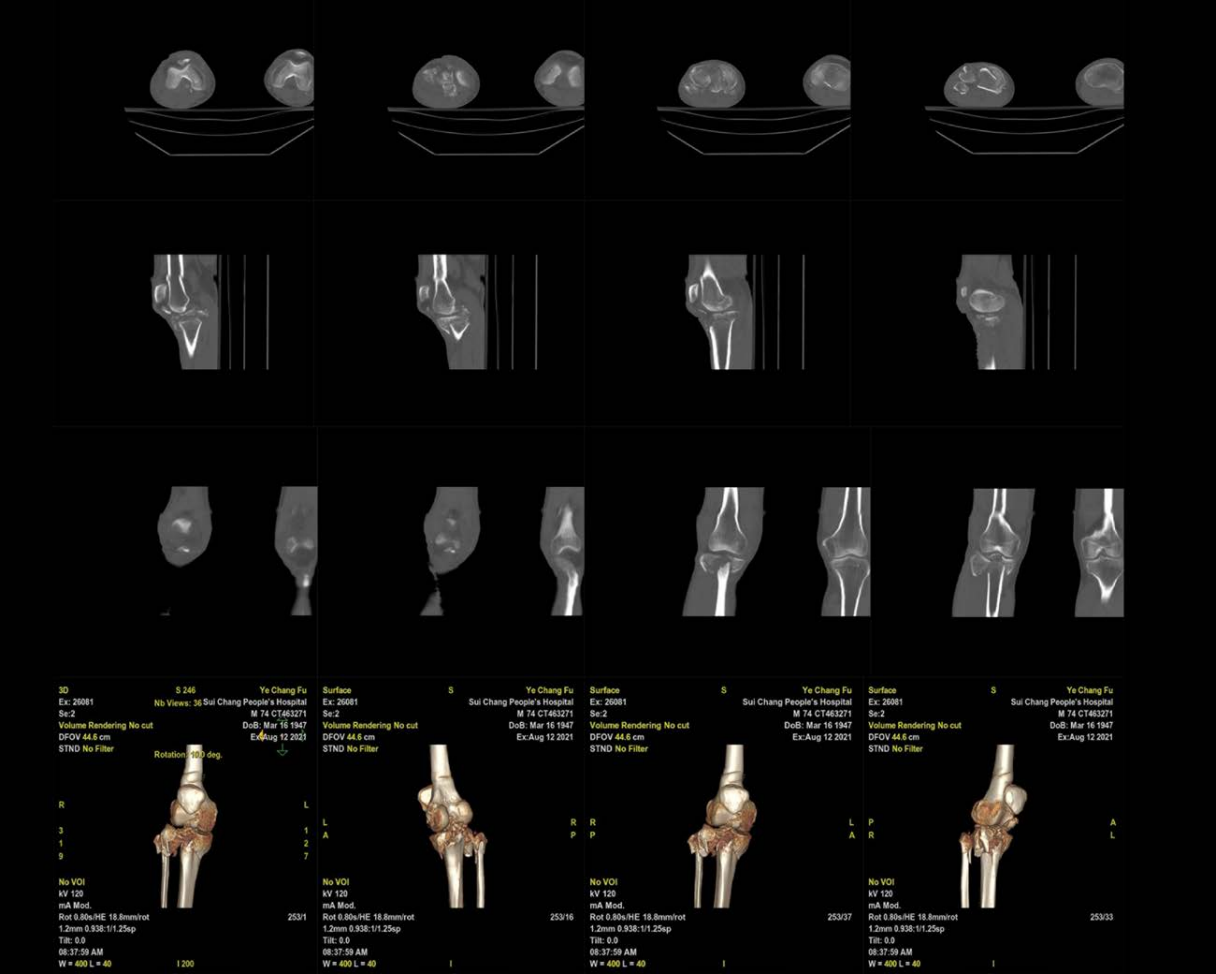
Computed tomography (CT) (Fig. 2; date of examination: August 12, 2021) revealed a clear traumatic hepatic rupture. Axial (A; B; C; D), agittal (E; F; G; H), coronal (I; J; K; L), and 3-dimensional (M; N; O; P) CT.
